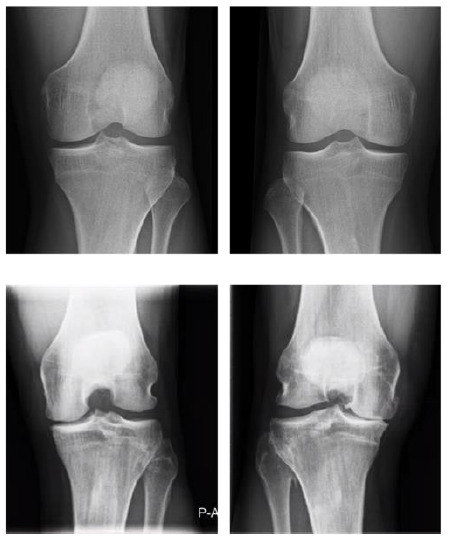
Knee radiographs (Rosenberg view) of two retired professional footballers (top two radiographs: Grade 0; bottom two radiographs: Grade 3–4). The bottom two radiographs demonstrate radiological features of OA including loss of joint space and osteophytes.
